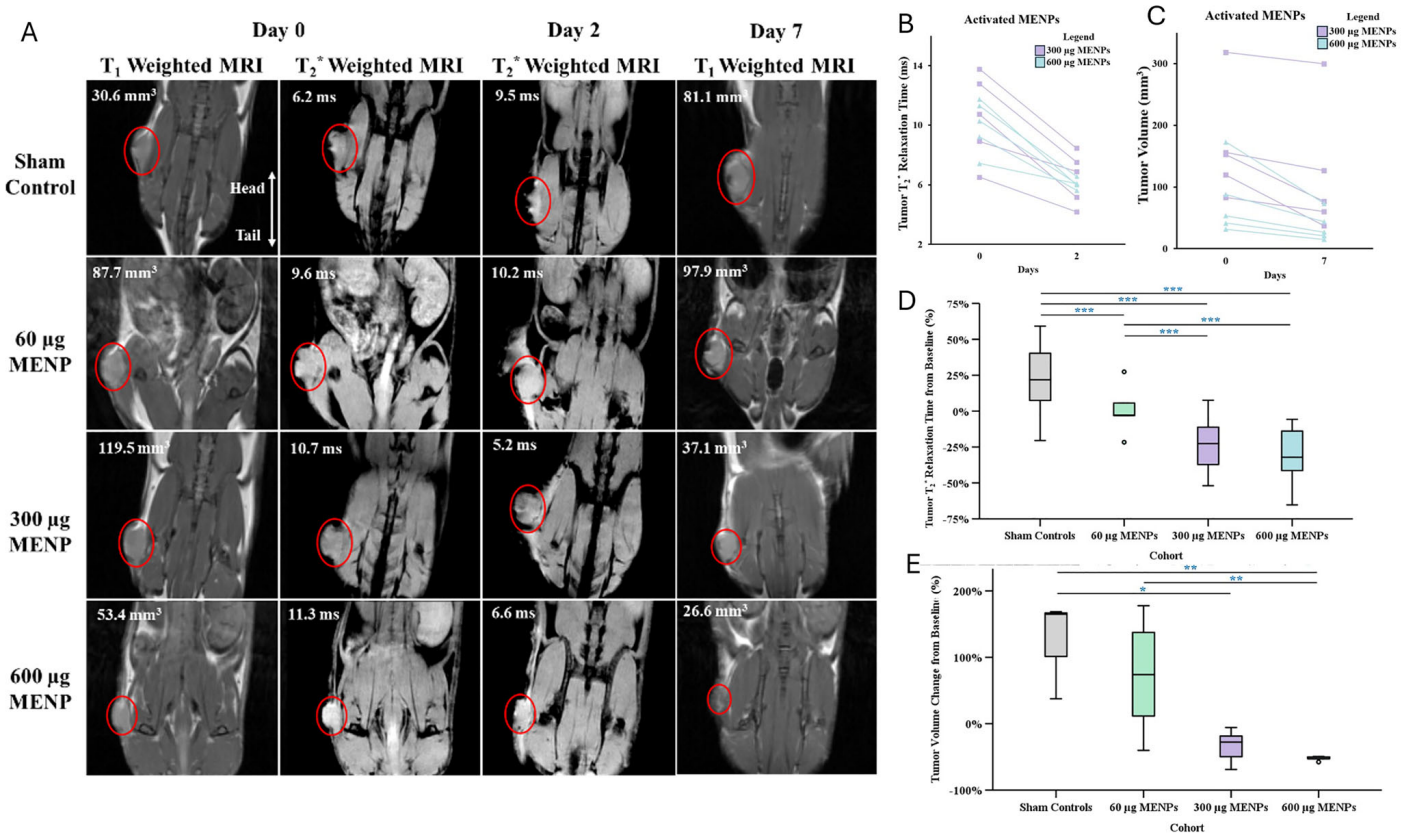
Tumor volume and relaxation time outcomes. A) Representative MRI images across cohorts at Days 0, 2, and 7. Images display T_1_‐weighted and T_2_
